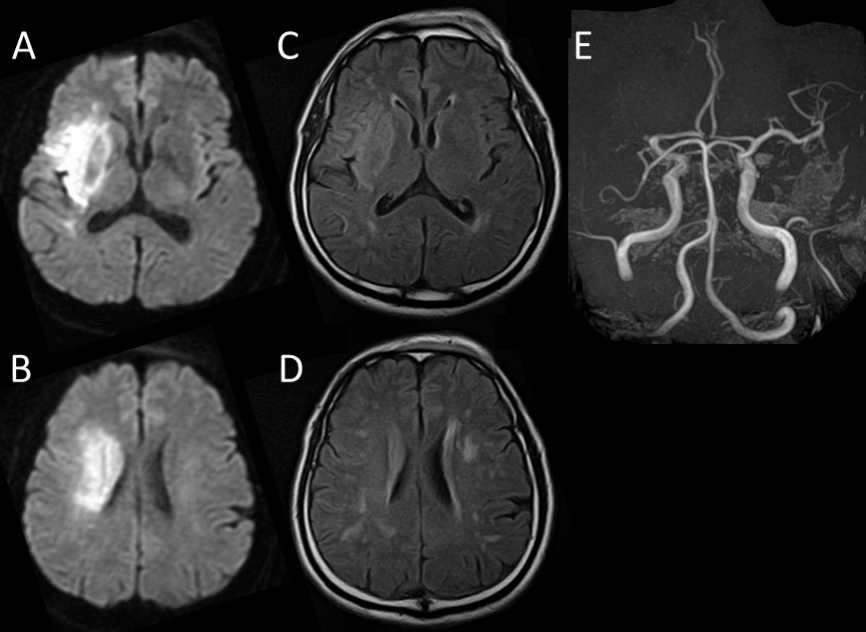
Initial magnetic resonance imaging A, B: diffusion-weighted imaging, C, D: fluid-attenuated inversion recovery and magnetic resonance angiography (E) acute infarction in the right middle cerebral artery (MCA) territory and MCA occlusion.
